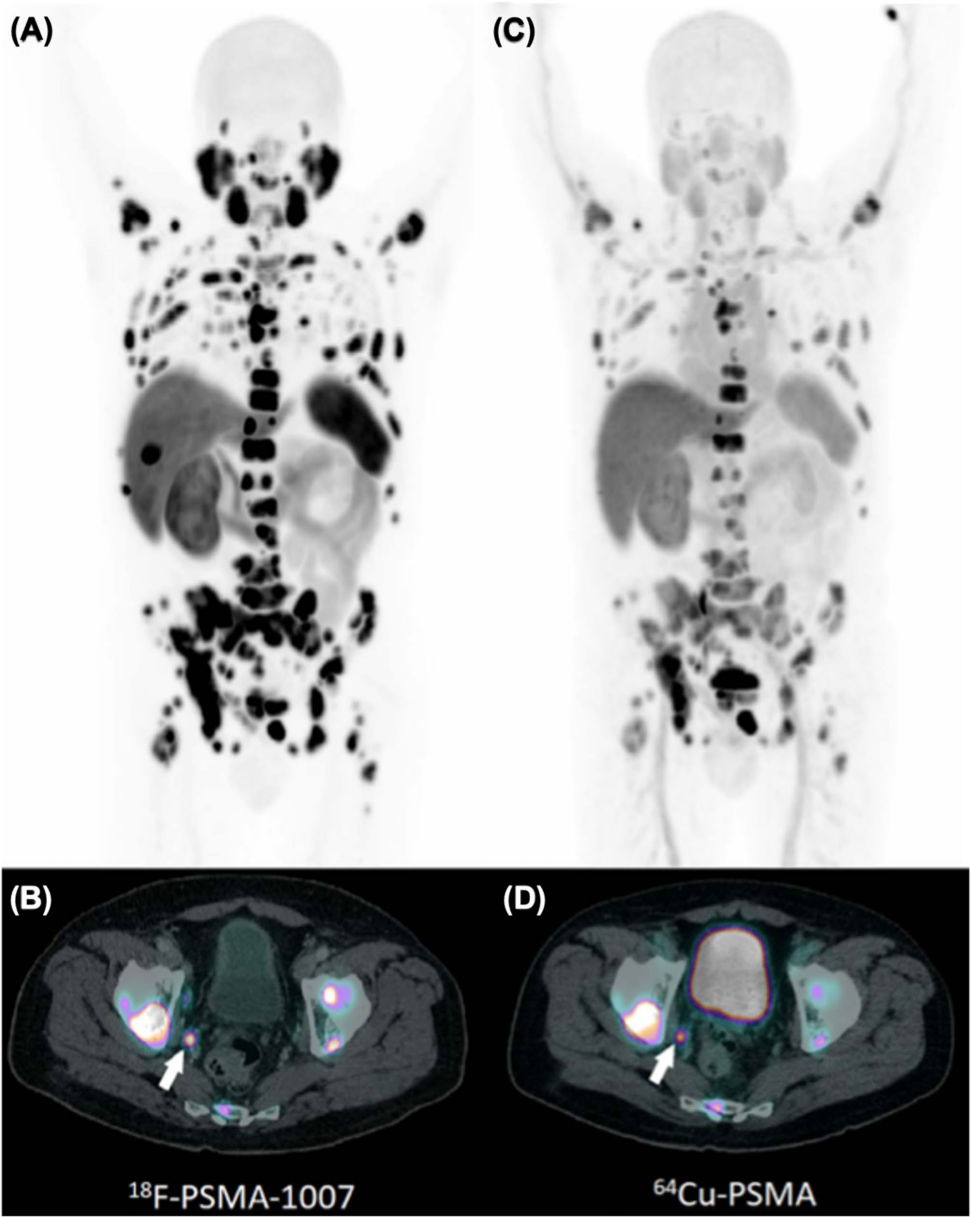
Comparison of PSMA-targeted PET/CT in metastatic PCa. Panels (A) (maximum-intensity projection) and (B) (fused axial PET/CT) show [^18^F]PSMA-1007 images of a 68-year-old man (Gleason score 9; PSA 1044 ng/mL). Panels (C) (maximum-intensity projection) and (D) (fused axial PET/CT) display corresponding [^64^Cu]Cu-PSMA images, revealing tracer uptake in identical skeletal metastases. High uptake in a right internal iliac lymph node is indicated by arrows in panels (B) and (D). Reproduced with permission from Cardoza-Ochoa et al. [101]
